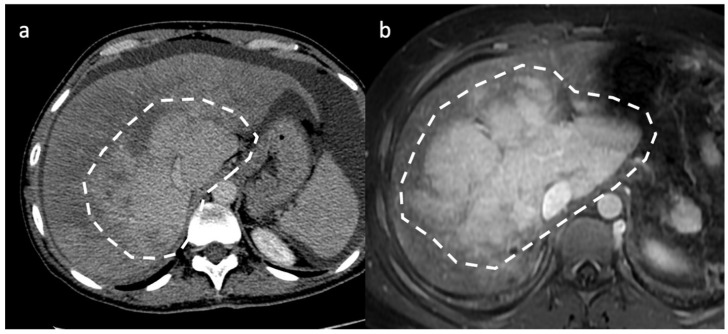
Two different cases of acute/subacute BCS. On axial portal venous phase CT (a) and MR (b) images the liver exhibits decreased peripheral enhancement caused by portal and sinusoidal stasis, with stronger central liver enhancement, a pattern called “zonal enhancement” (white circles).
